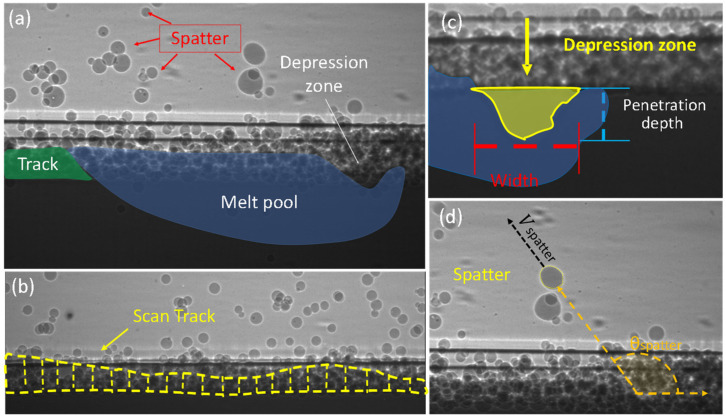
Selective laser melting dynamics. (a) A representative X-ray image acquired by high-speed X-ray imaging with depression zone, melt pool, spatter, and scan track highlighted. (b) Post-scan X-ray image with the location and geometry of melted scan track marked. (c) X-ray image with depression zone geometry indicated. (d) X-ray image with the spatter direction $\theta_{spatter}$ and velocity $V_{spatter}$ indicated.
